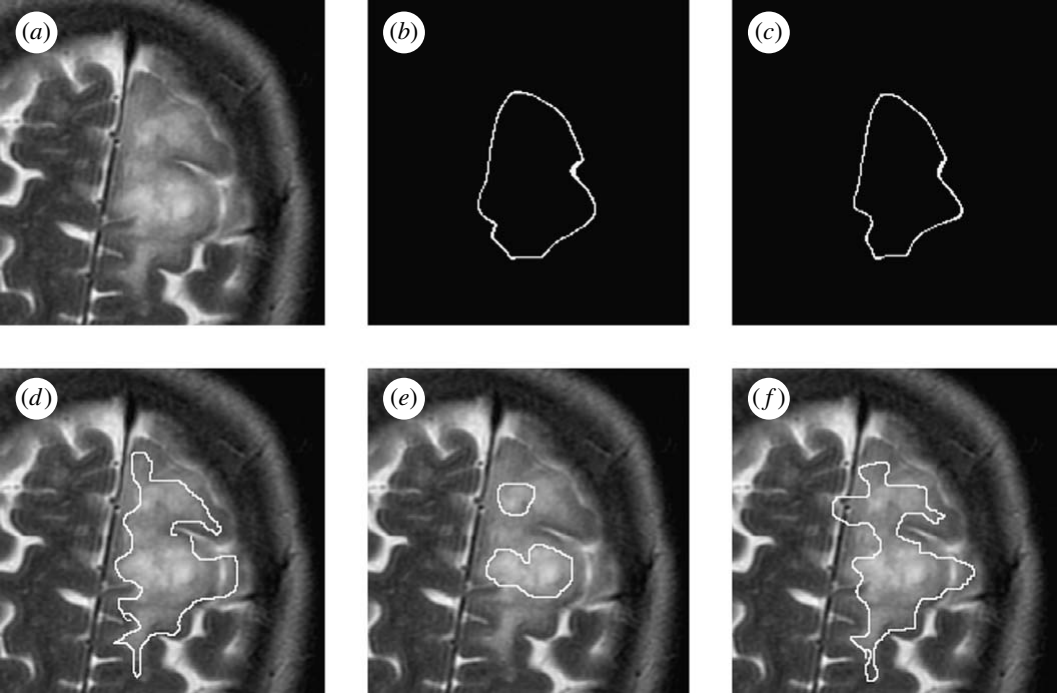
Estimated true segmentation from human rater segmentations. The estimated true contour is consistent with the human raters, and has a better spatial overlap with the rater segmentations, as measured by the Dice coefficient, than with the average segmentation. The best, worst and median rater segmentations as determined by the algorithm are also displayed. (*a*) MRI of a brain tumour. (*b*) Average contour. (*c*) Estimated true contour. (*d*) Best rater segmentation. (*e*) Worst rater segmentation. (*f*) Median rater segmentation.
